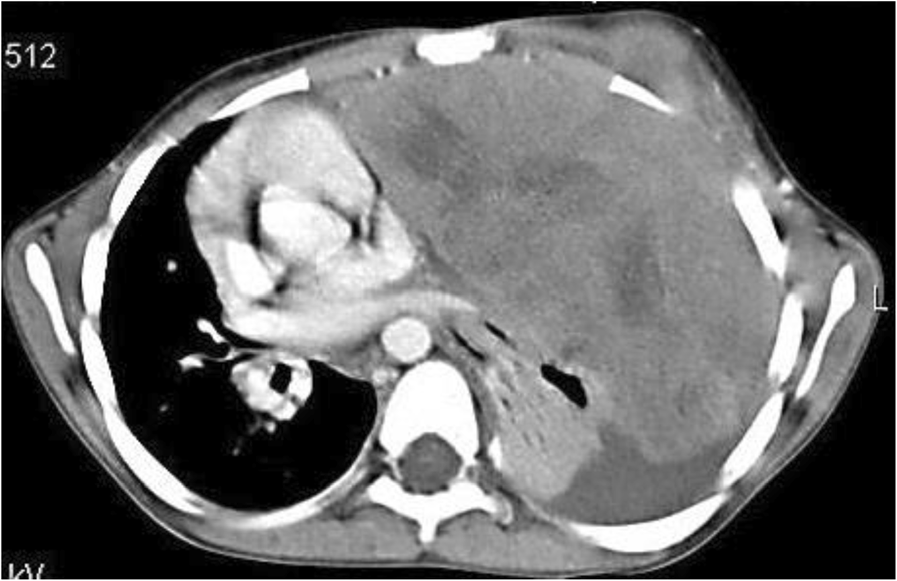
CT scan of a 10-year-old girl revealed a large lesion (mean diameter 16 cm) in the left hemithorax
